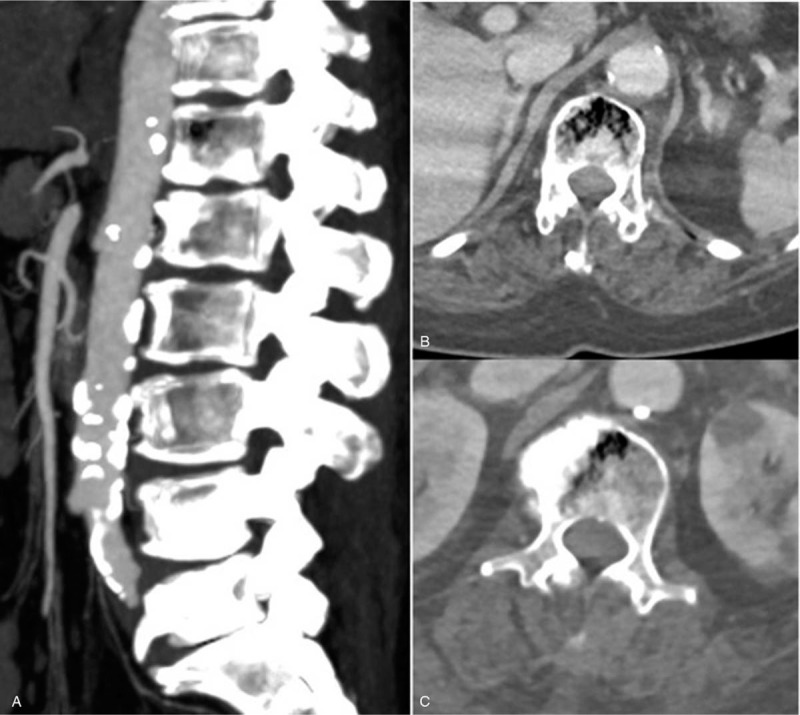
Chest and abdominal computed tomography scan at the time of admission showing intraosseous mottled air in the T12 (B) and L1 (C) vertebral bodies.
